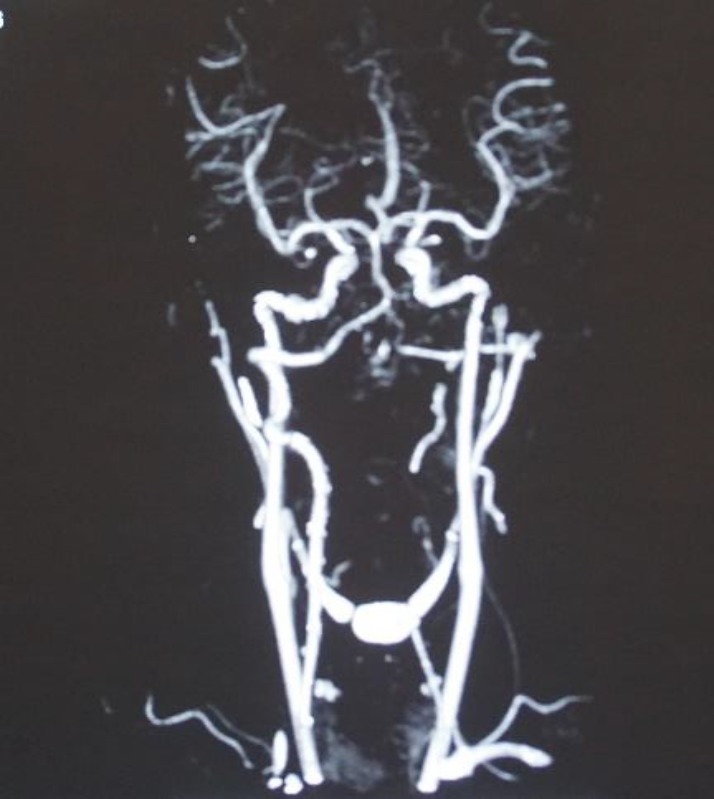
CT angiography revealed the absence of definitive feeding vessel supplying the tumor
